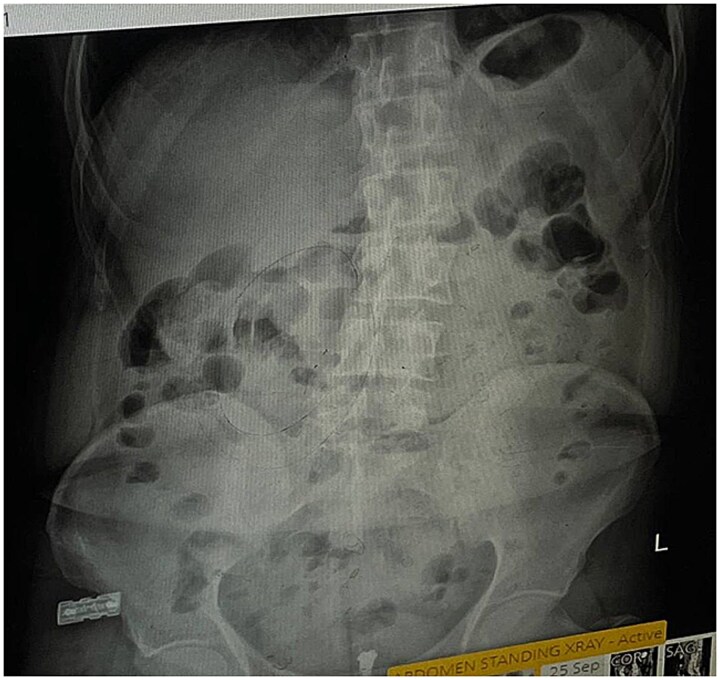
Erect abdominal X-ray demonstrating multiple dilated bowel loops with multiple air–fluid levels, consistent with intestinal obstruction.
